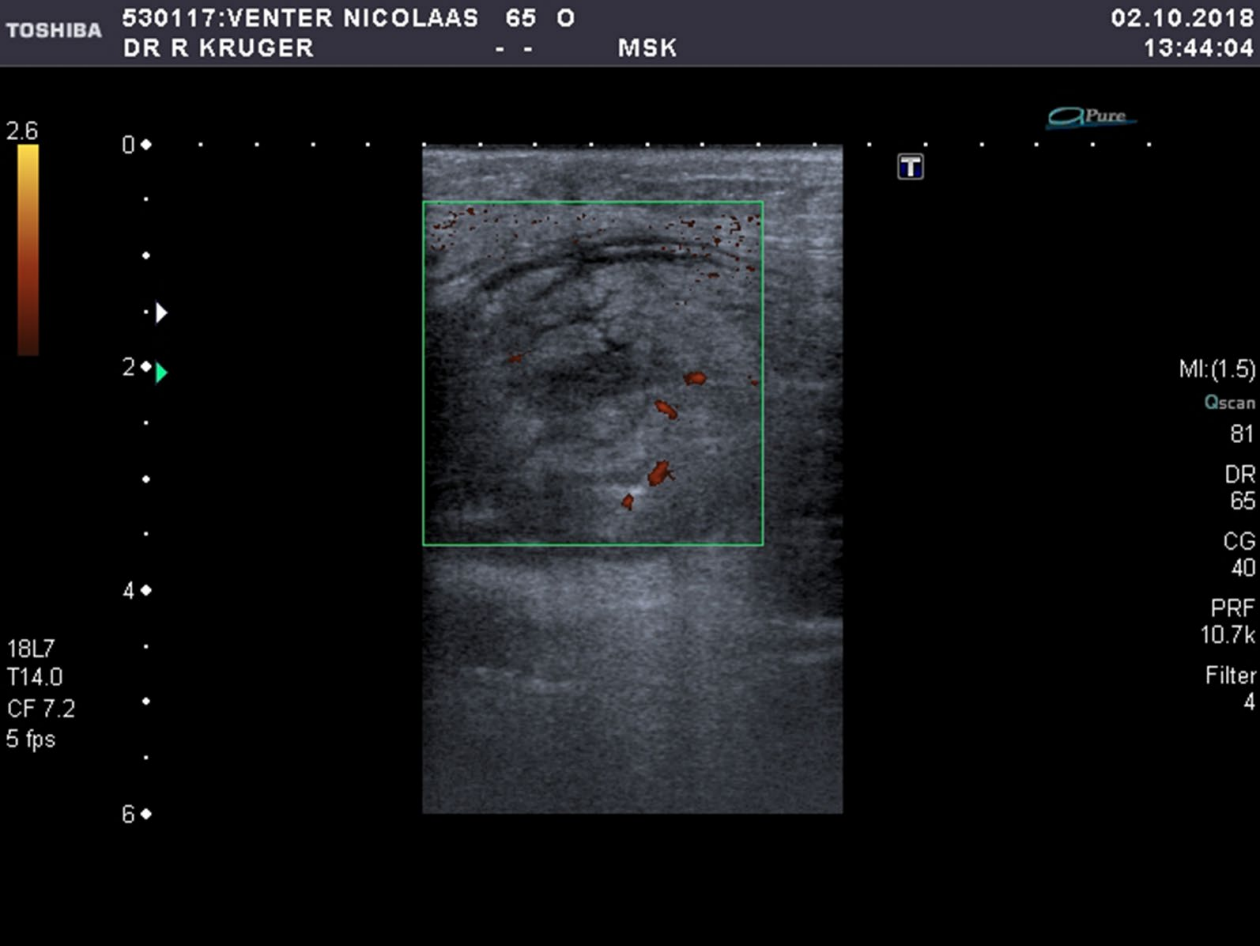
Increased vascularity noted with Power Doppler imaging
